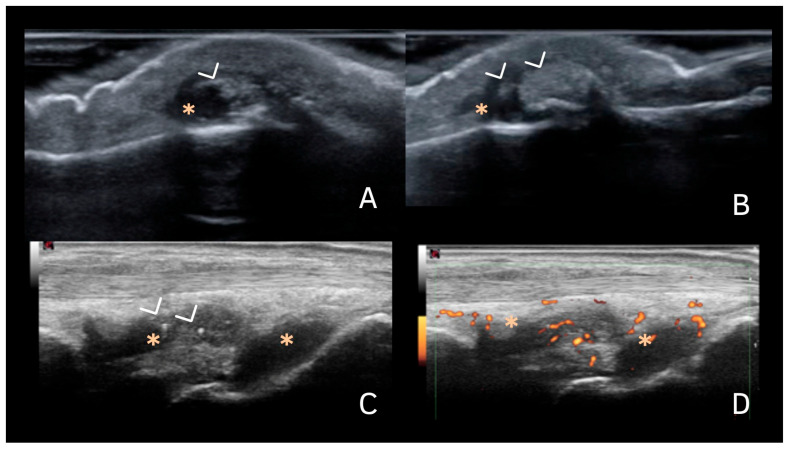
Synovial deposits of CPP. (A,B)—Longitudinal scan of distal interphalangeal joints, showing CPP deposits in synovial tissue; (*) synovitis; (arrowhead) CPP crystals. (C,D)—Longitudinal view of the wrist showing punctate hyperechoic synovial deposits and active synovitis with positive Power Doppler signal in a patient presenting with pseudogout phenotype. Source: personal files.
